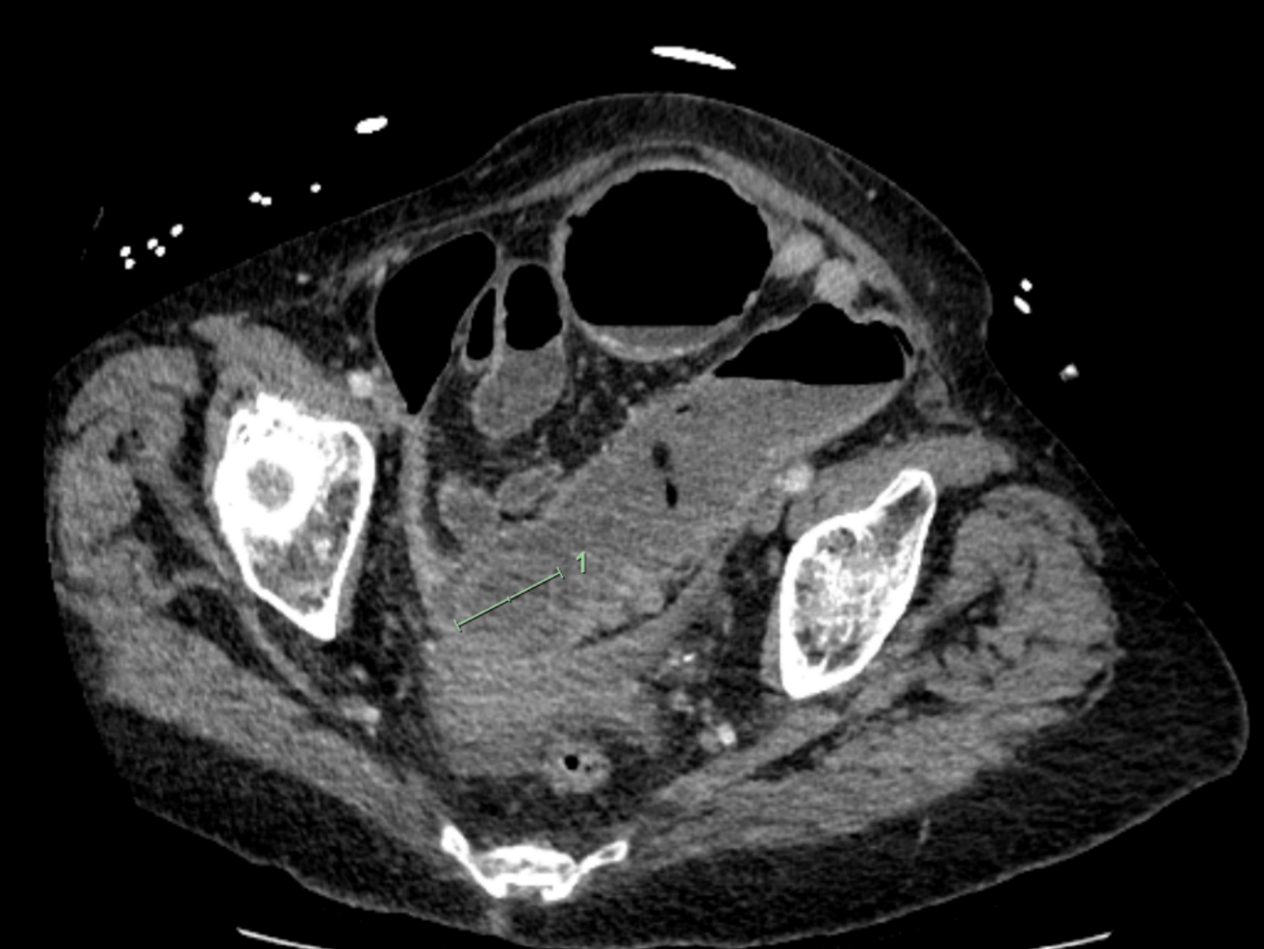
Abdominal CT scan: coronal view showing this impacted gallstone with a dilated large bowel
